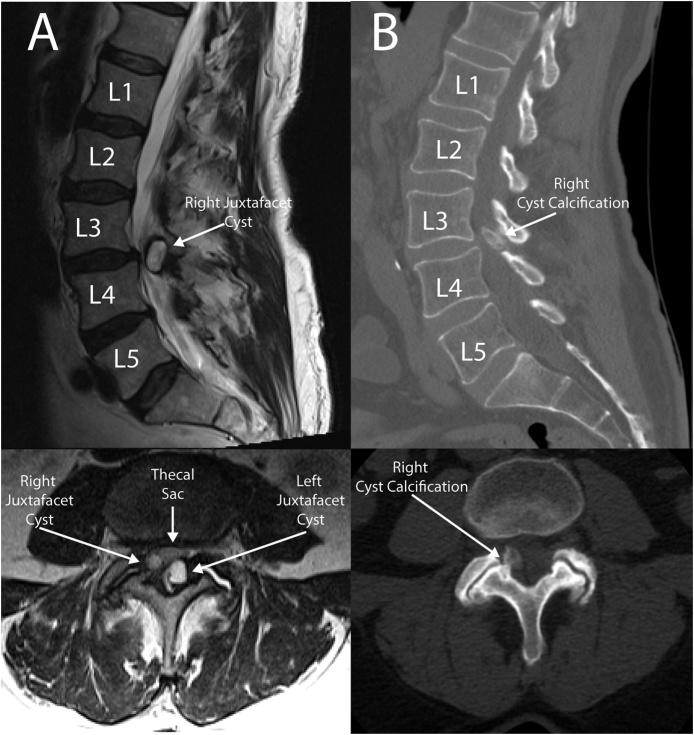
A) Pre-operative MRI (sagittal-top, axial-bottom) demonstrates bilateral juxtafacet cysts (left > right). B) Pre-operative CT (sagittal-top, axial-bottom) shows calcification of the smaller right-sided cyst.
